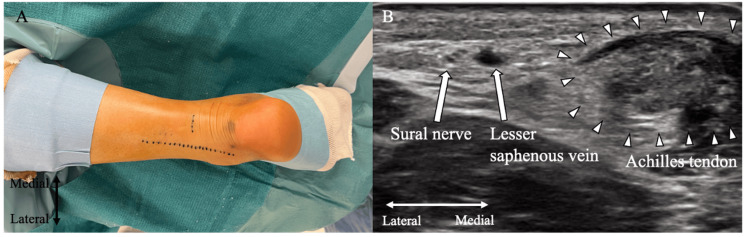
Ultrasound-guided preoperative marking of the sural nerve A: Sural nerve pathway; B: Ultrasound visualization of the sural nerve Image source: Authors
